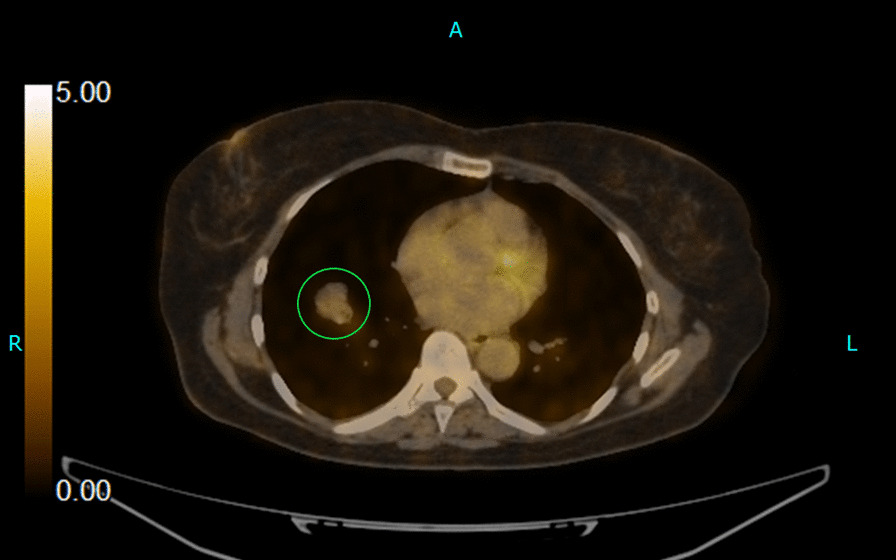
FDG PET/CT image; Lobulated soft tissue lesion in the right lung encircled in green
